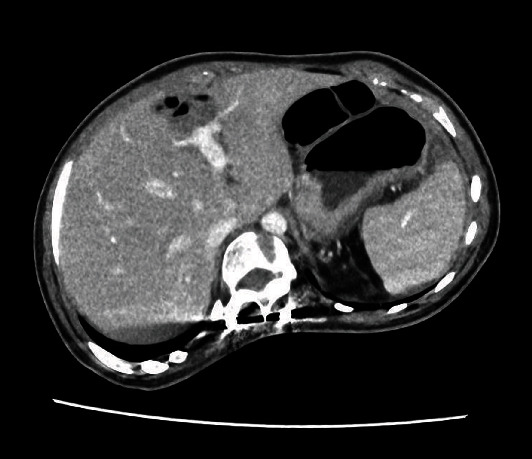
Postoperative CT scan (2 months).
